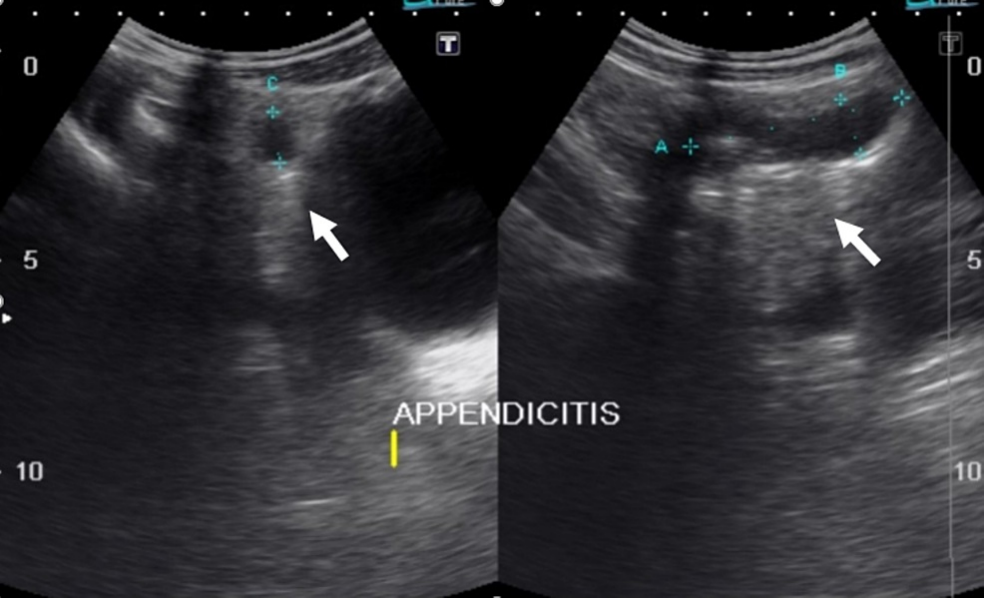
Grade 3 PFES. 13 years male presented with lower abdominal pain and vomiting. Ultrasound of the right iliac fossa reveals a dilated blind-ending bowel loop in RIF measuring 5 centimeters in length (A) and 1.2 centimeters in short axis (B) representing acute appendicitis. An appendicolith also noted within the appendicular lumen. Periappendiceal fat echo (white arrow) is so echogenic that the underlying structures are not visualized consistently with grade 3 PFES (periappendiceal fat echo sign).
